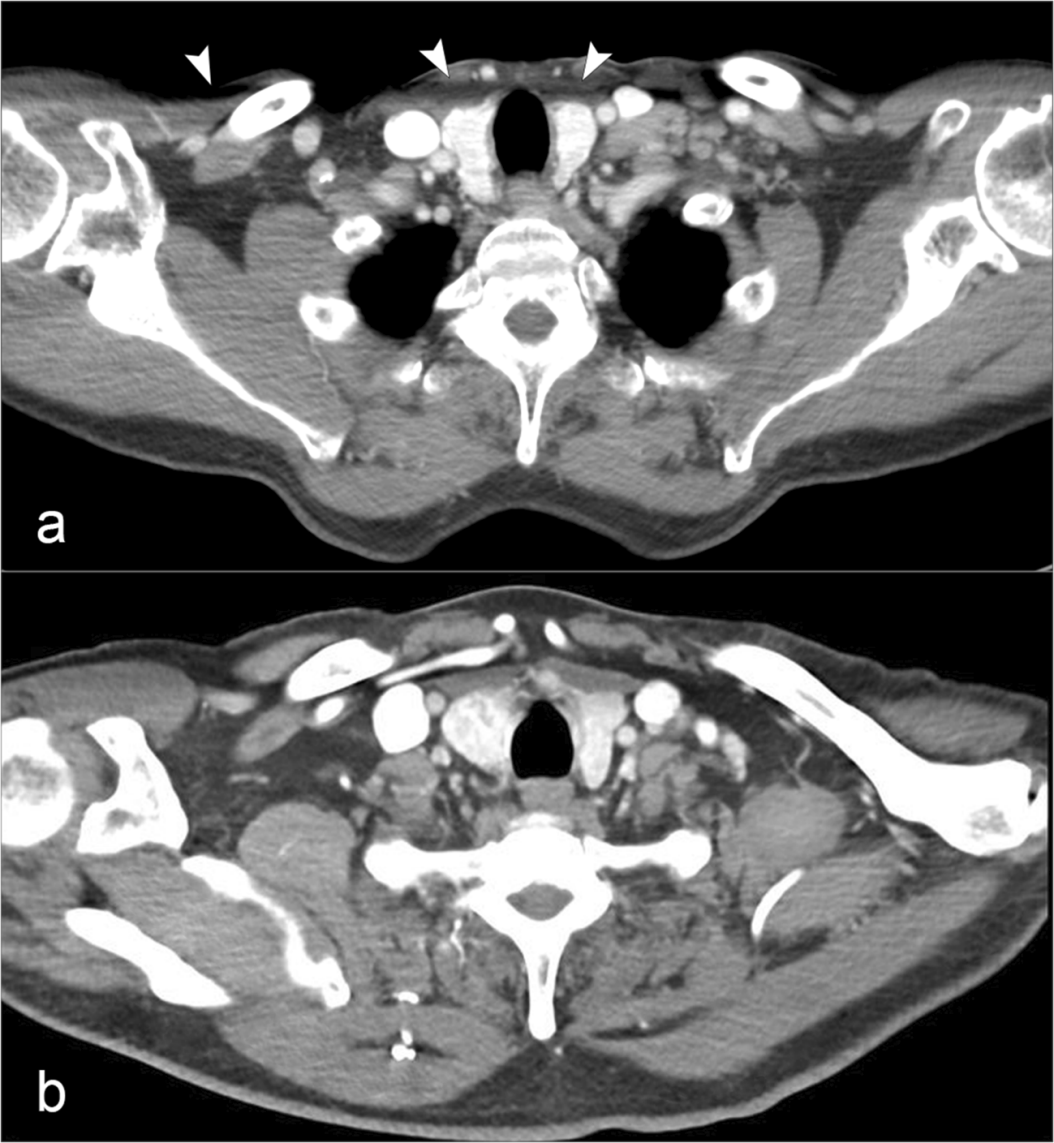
Streak artifacts at the cervicothoracic transition zone in single-energy CT (SECT). Streak artifact (white arrows) found at the level of the shoulders with the SECT technique. Images are from a 63-year-old male patient with a cT2N0 carcinoma of the oropharynx who underwent SECT at 70 kV (a) and a 52-year-old male patient who underwent dual-energy CT (DECT) for staging completion (b). All images are shown in the same window setting (center = 50 HU, width = 500 HU). No streak artifacts were found with DECT at the height of the shoulders
